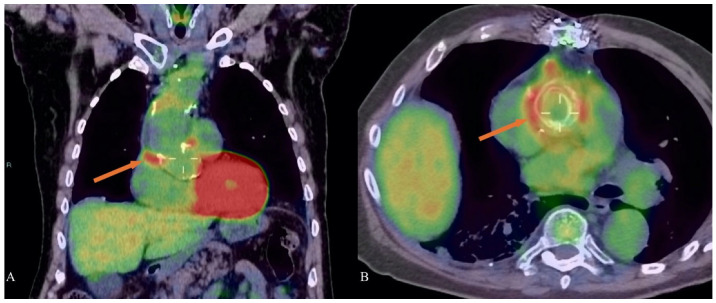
A case of aortic prosthetic graft infection in a patient with Marfan syndrome evaluated with ^18^F-fluorodeoxyglucose (^18^F-FDG) positron emission tomography/computed tomography (PET/CT): coronal (A) and axial (B) images show hypermetabolism around the aortic prosthetic graft (orange arrows) and site of infection process.
